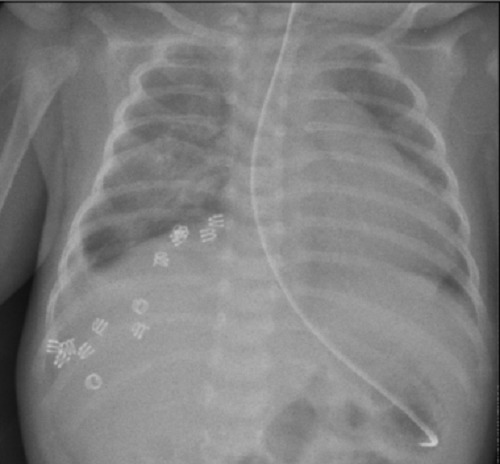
Figure 2: Chest X-ray. Post-operative spiral tack application
